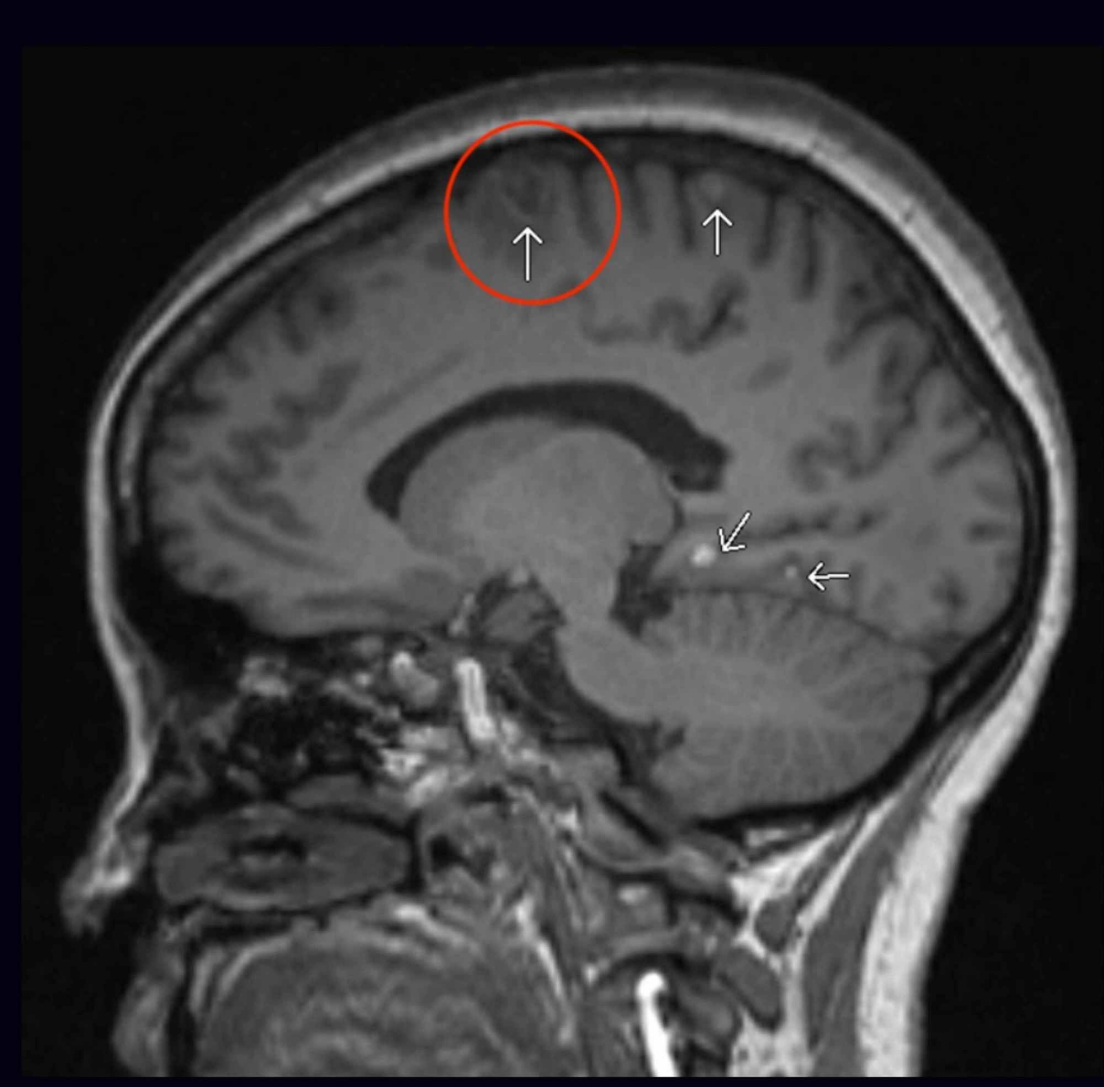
MRI brain sagittal view with numerous contrast-enhancing lesions (white arrows) within cerebral hemisphere measuring between 2-14 mm, the largest lesion being 12 x 13 x 14 mm within the superior left frontal lobe with mild surrounding vasogenic edema (red circle) MRI: magnetic resonance imaging; mm: millimeter
